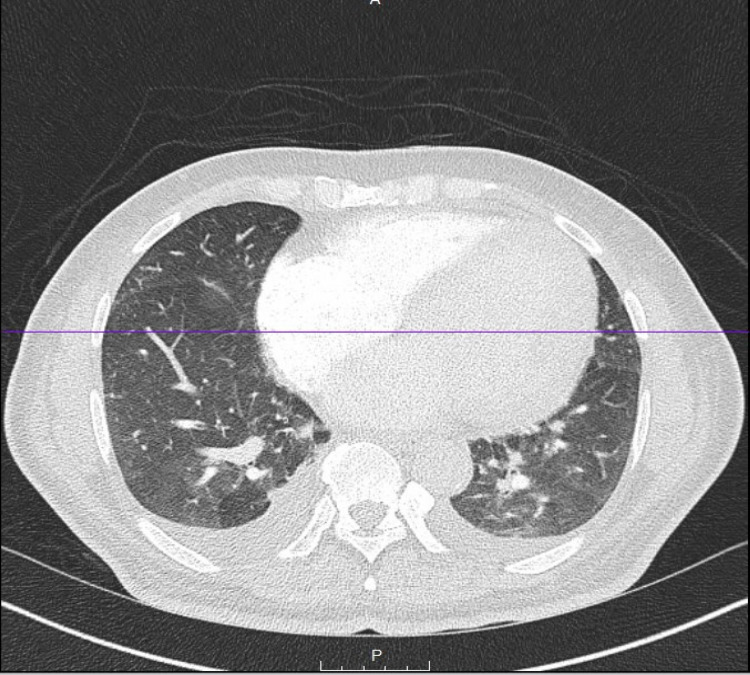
Chest CT angiogram (CTA)
